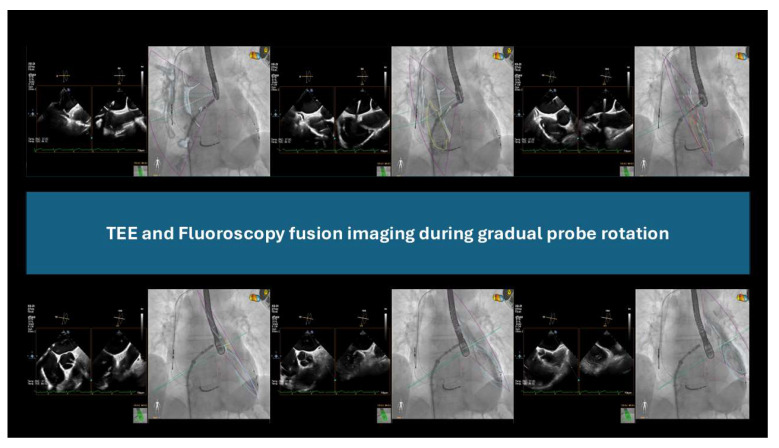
Transesophageal echocardiography and fluoroscopy fusion imaging.
